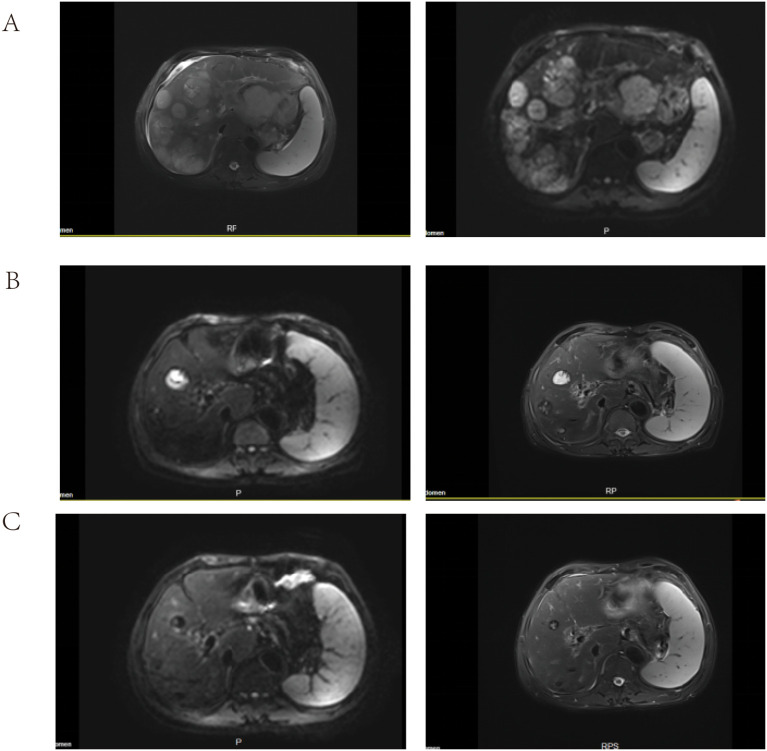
Liver MRI demonstrating treatment response over time. (A) April 7, 2024 (baseline) shows multiple hepatic metastases. (B) June 7, 2024, during FOLFOX plus serplulimab, shows interval shrinkage of target lesions. (C) Sept 16, 2024 shows continued reduction without new lesions.
